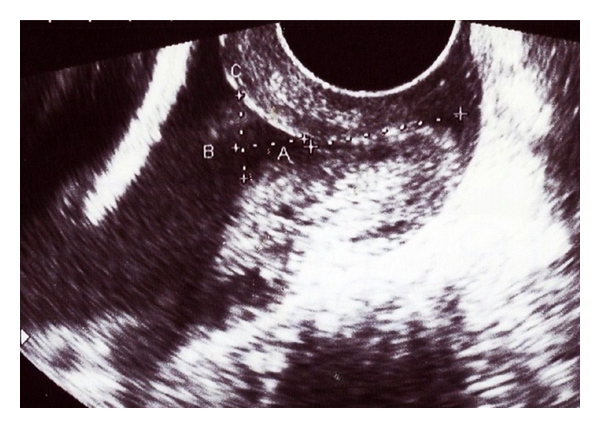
Transvaginal ultrasound image of the cervical funneling.
